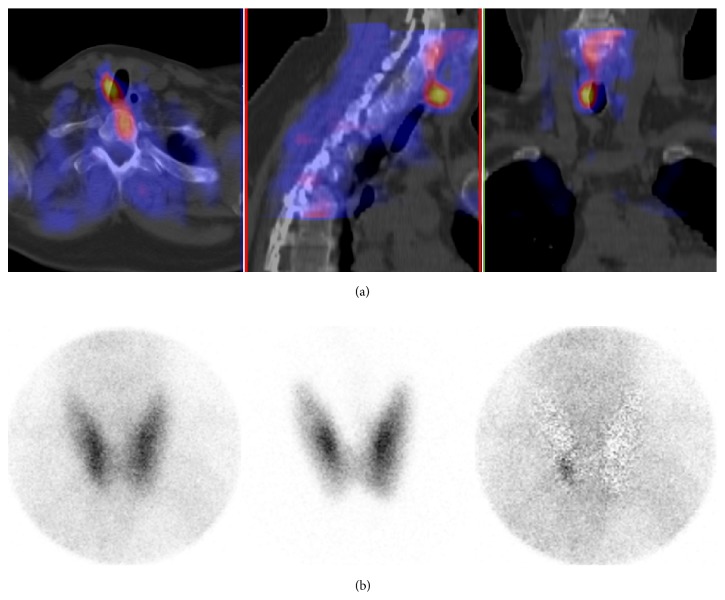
An example of a true-positive finding with SPECT/CT and with pinhole in primary hyperparathyroidism. (a) Fusion SPECT/CT images. (b) Anterior pinhole images (*left*: ^99m^Tc-sestamibi image;* middle*: ^123^I image;* right*: subtraction image). A 230 mg adenoma (arrows) was resected behind the lower right quadrant of the thyroid.
